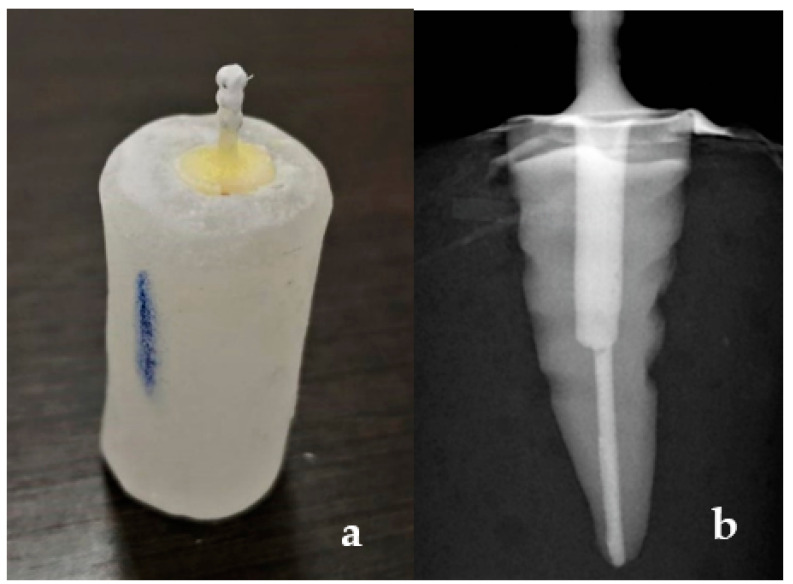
(a) Cemented fiber post; (b) Radiograph after the cementation of post.
